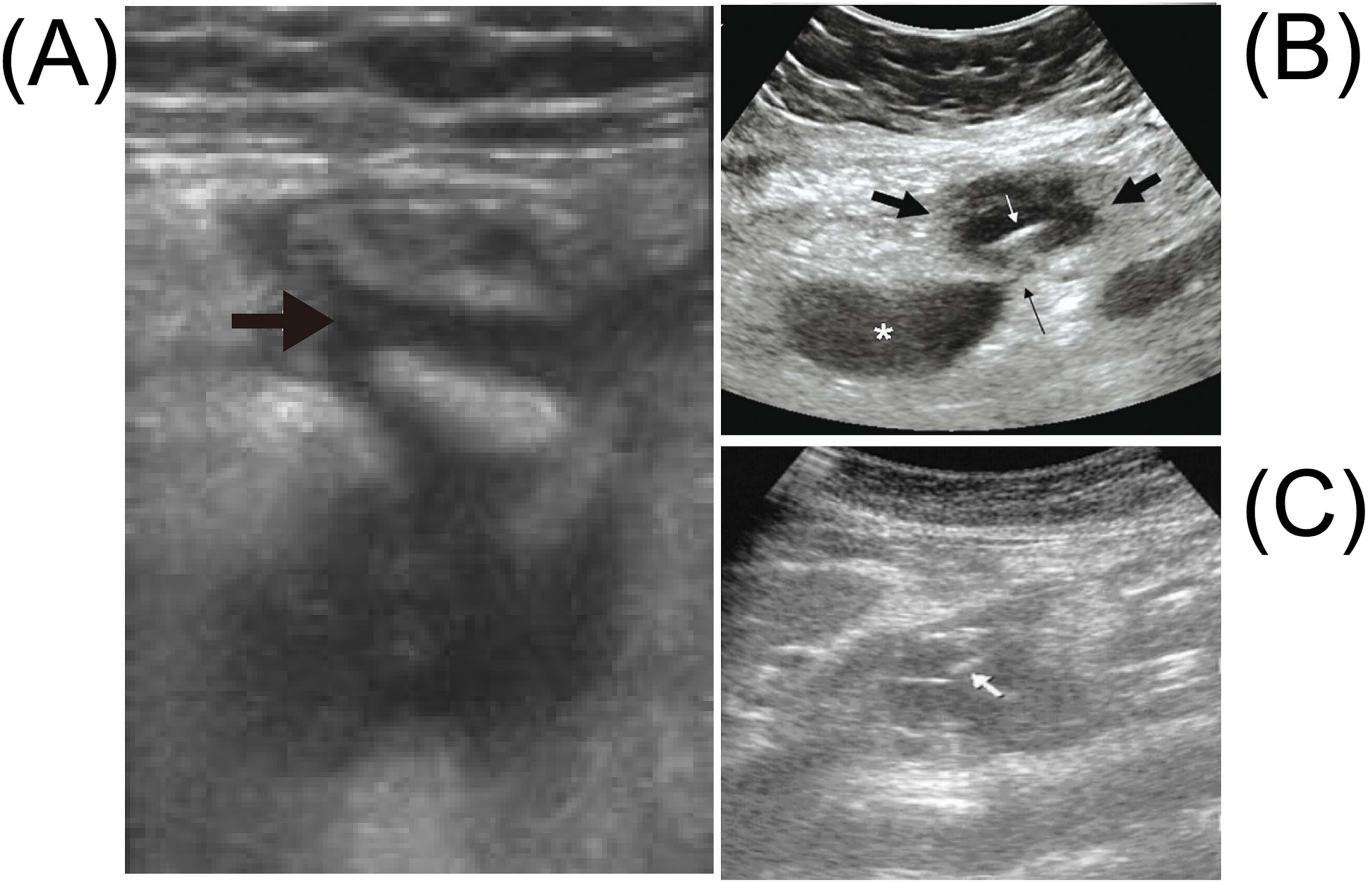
Abdominal ultrasound for intestinal perforation caused by a fishbone shows the following. (A) The abdominal ultrasound examination reveals fluid accumulation in the right lower abdomen (black arrow). Adapted from reference (29) with permission from Elsevier. (B) The transverse sonographic image of the right periumbilical region shows a hypoechoic mass with a maximum diameter of 4.5 cm (thick black arrow), containing a thin linear echogenic structure measuring 3.7 cm in length (white arrow), representing the fishbone. There is also a hypoechoic sentinel loop of small intestine (asterisk) adjacent to the mass without peristalsis, connected to it via a 2-mm-wide linear hypoechoic sinus tract (thin black arrow). Note the intense echogenicity of the omental fat surrounding the lesion. Adapted from reference (50) with permission from Galenos. (C) The abdominal ultrasound shows a hypoechoic mass around the pancreas, with linear echogenic structures around the pancreas (white arrow). Adapted from reference (142) with permission from Oxford Academic.
